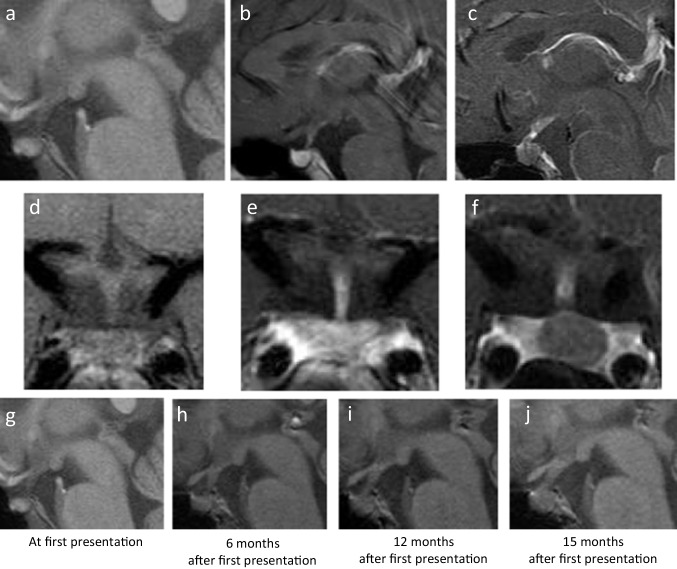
MRI images of pituitary lesion in patient 1. Sagittal (a) and coronal (d) plain T1-weighted images at first presentation. Sagittal (b) and coronal (e) gadolinium-enhanced T1-weighted images at first presentation. Sagittal (c) and coronal (f) gadolinium-enhanced T1-weighted images 15 months after first presentation. Serial sagittal plain T1-weighted images (g–j). At first presentation (g), and 6 (h), 12 (i), and 15 (j) months after first presentation. MRI, magnetic resonance imaging
